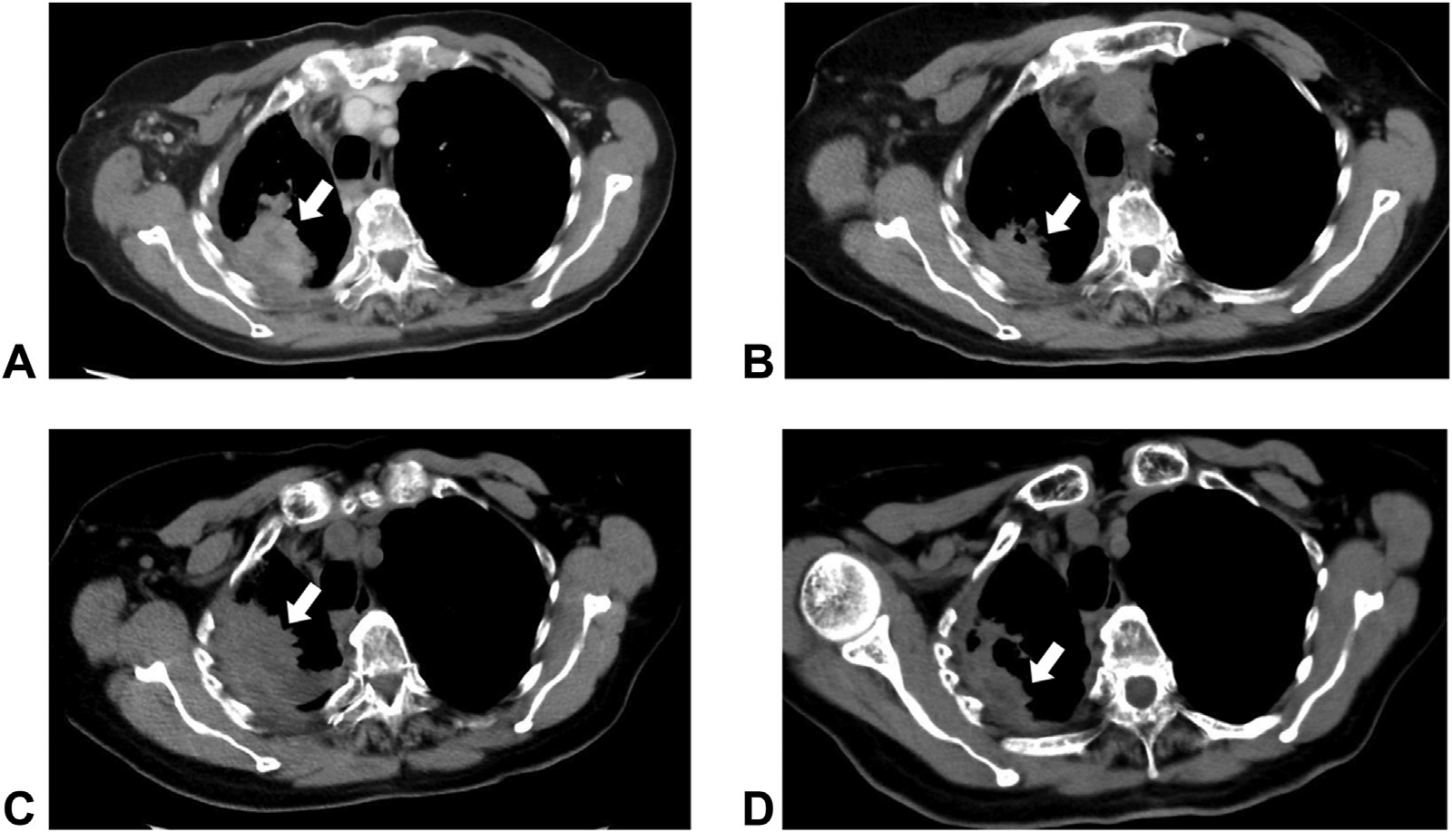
Computed tomography images of the tumor in the right upper lobe of the lung. (A) Before the start of entrectinib treatment. (B) Three months after initiation of entrectinib treatment revealing a size reduction. (C) Before crizotinib treatment. (D) Two months after initiation of crizotinib treatment revealing tumor shrinkage. White arrows indicate the lesions.
